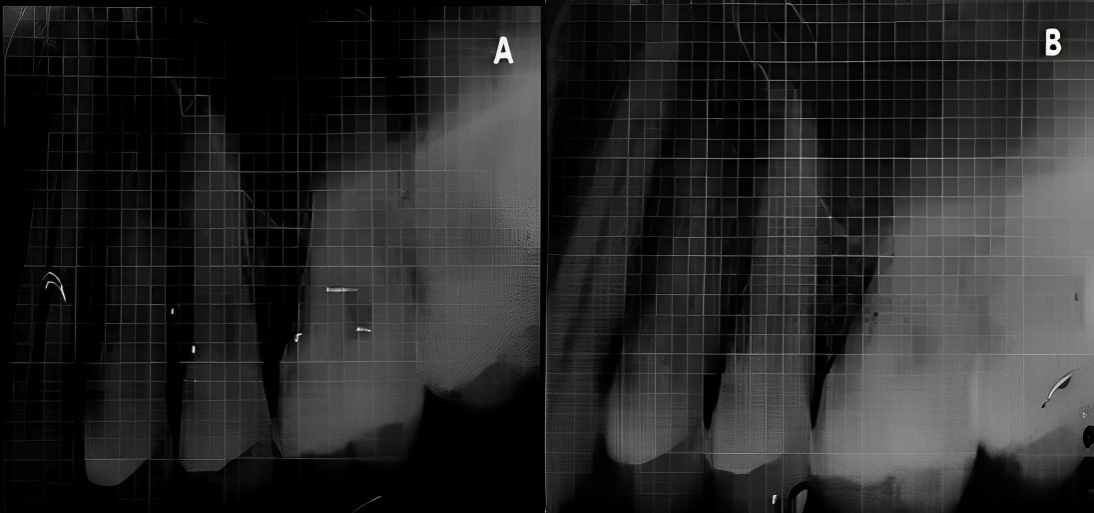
Clinical parameters that were evaluated included plaque index (PI), gingival bleeding index (GBI), PPD, and CAL. The evaluated radiographic parameters included bone fill (BF), bone fill percentage (BF%), bone crest change (BCC), and bone crest change percentage (BCC%). The parameters were measured based on the radiographic changes at the base of the defect (BD) and alveolar crest (AC) from the cementoenamel junction (CEJ) and correction factor (CF) as follows.^10,11^
